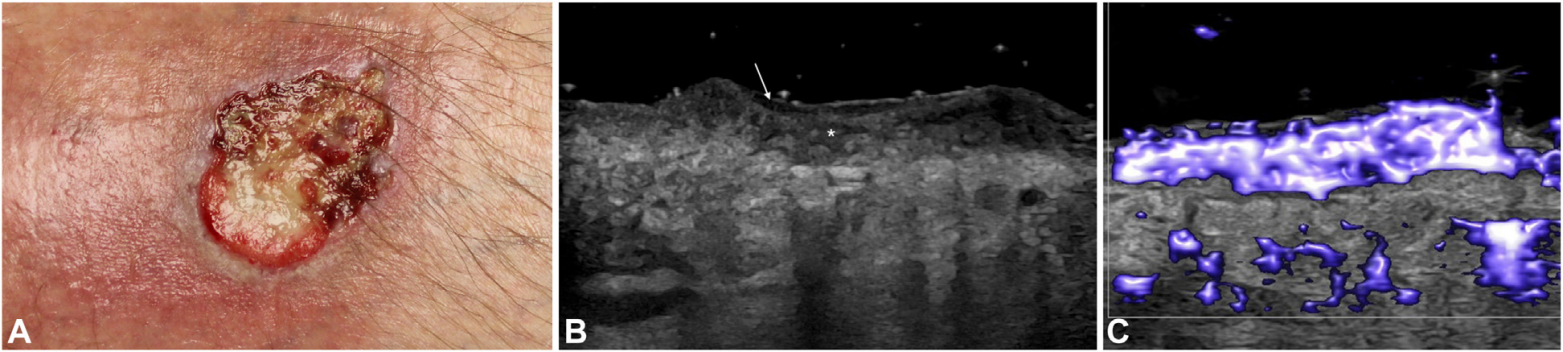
A, Basal cell carcinoma (PG-mimicking ulceration) on right lower extremity. B, HFUS demonstrates shallow heterogenous wound bed (∗) and hyperehoic epidermis seen over entire wound with underlying hypoechoic layer (*arrow*). C, HFUS microvascular imaging with extensive hyperemia in wound bed involving epidermis layer. *HFUS*, High frequency ultrasound; *PG*, pyoderma gangrenosum.
